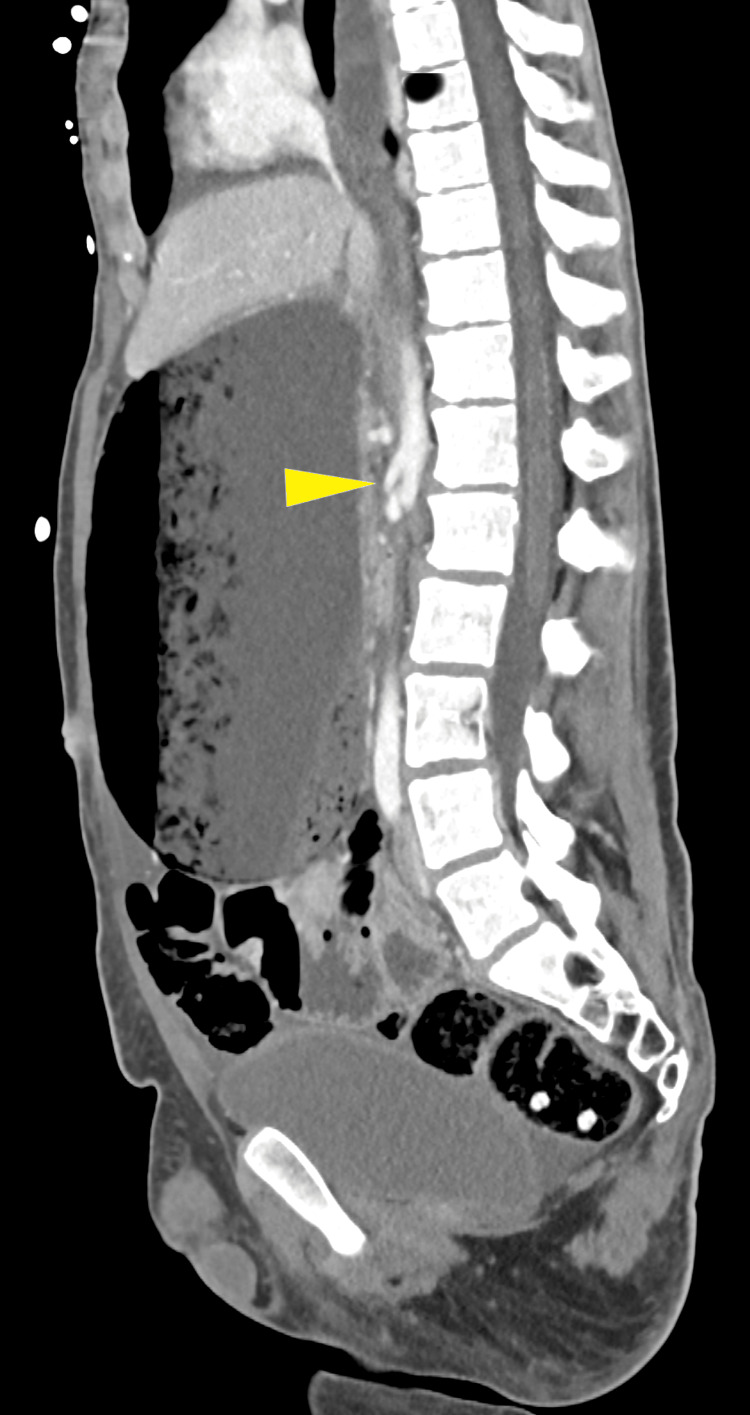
Sagittal view of the abdominal CT scan showing a narrowed AM angle. Yellow arrow: aortomesenteric angle. CT: computed tomography; AM: aortomesenteric
